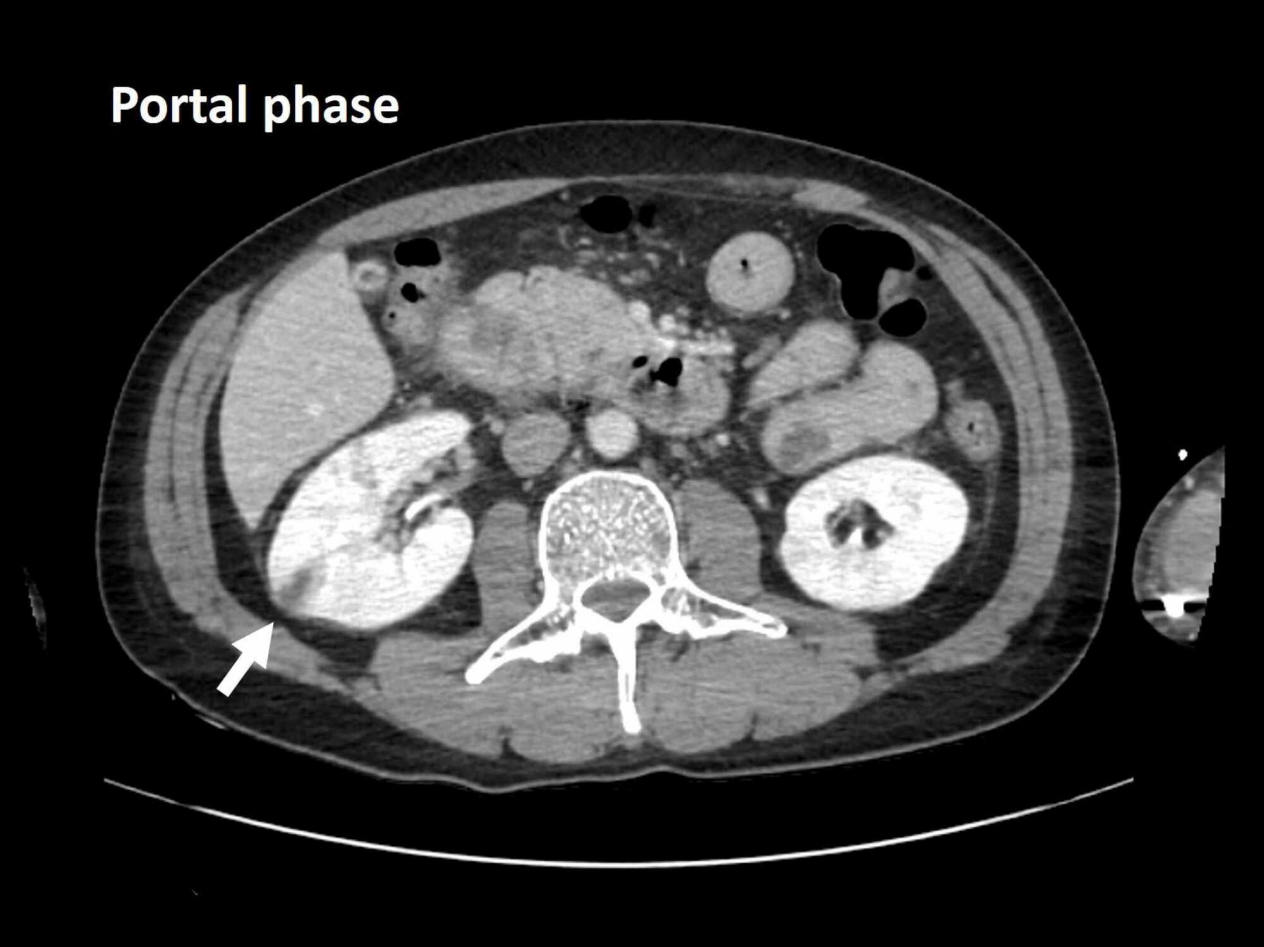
Initial contrast-enhanced computed tomography images of the chest and abdomen The computed tomography images showing a wedge-shaped area in the right kidney (white arrow).
